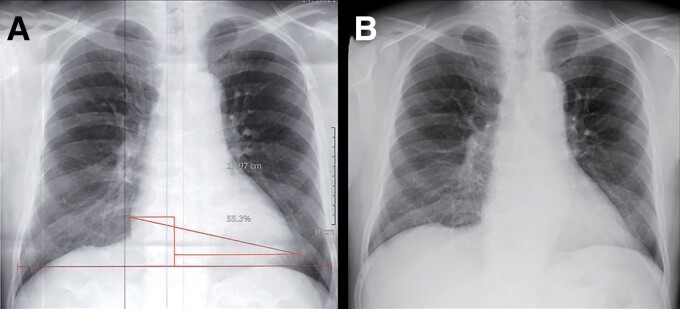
The chest X-ray before (*A*) and 3 months after (*B*) the introduction of continuous positive airway pressure therapy.
